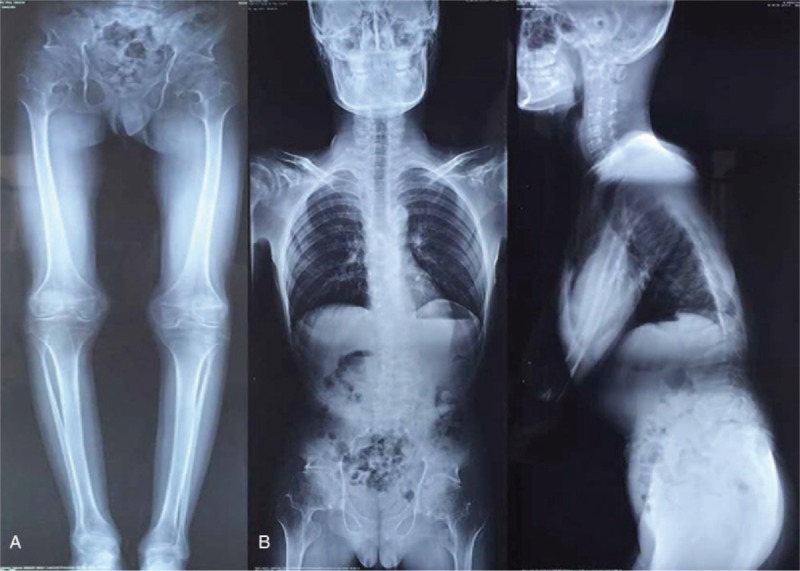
(A) Radiograph showed broadening width of the pubic symphysis, enlargement of femoral heads, narrowing hip joint space, enlargement of femoral condylar and narrowed joint space of knees. (B) Spinal X-rays showed platyspondyly, irregular end plates, and mild scoliosis.
